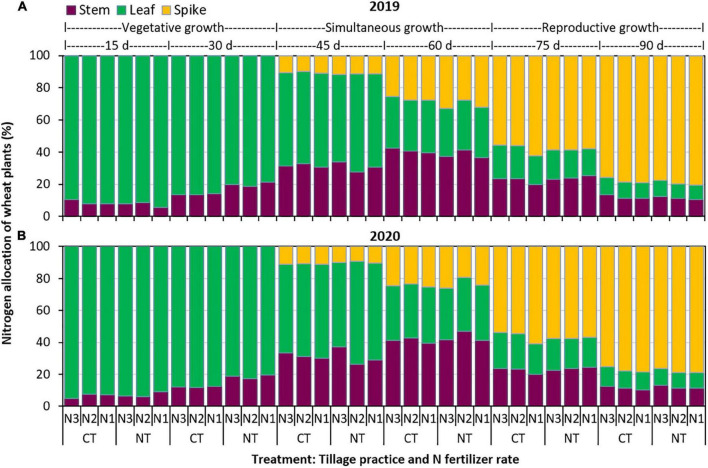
Nitrogen allocation (NA) in the stem, leaf, and spike of wheat plants with two tillage practices and three N fertilizer rates in (A) 2019 and (B) 2020. CT and NT represent conventional tillage without plastic film mulching and no-till with previous plastic film mulching. N1, N2, and N3 represent N fertilizer applied at 135, 180, and 225 kg N ha^– 1^, respectively.
